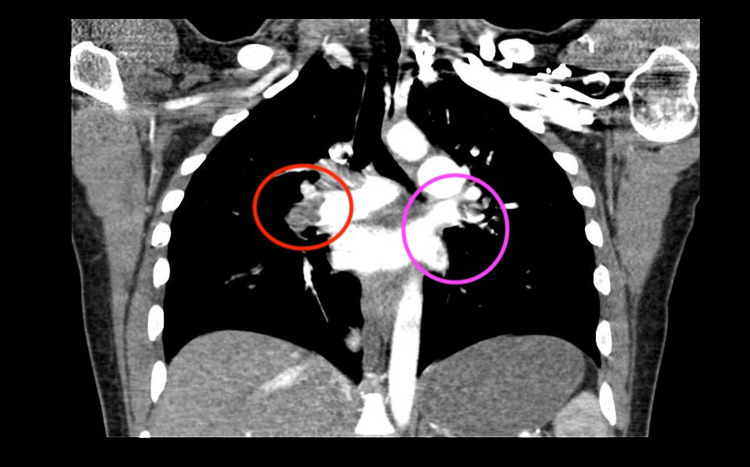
A CT angiogram of the chest (coronal) shows filling defects in the right pulmonary artery (red circle) and filling defects in the left pulmonary artery (pink circle), indicating bilateral pulmonary emboli.
